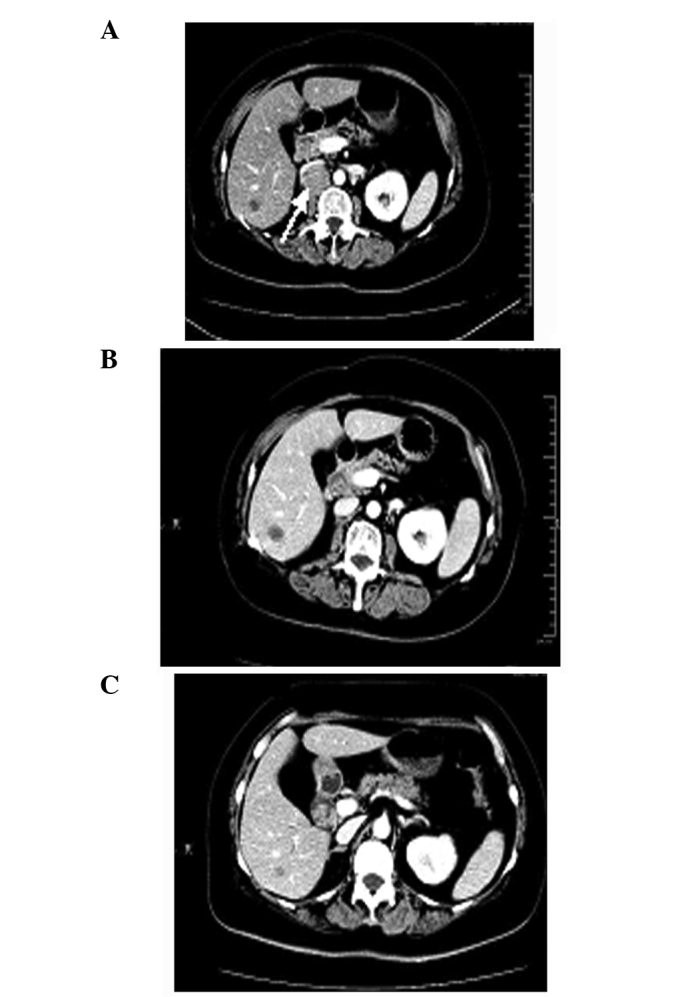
CT scan revealing (A) local recurrences in the retroperitoneum and (B) a considerably smaller tumor following chemotherapy with 80 mg/m^2^ intravenous irinotecan on days 1 and 8 and 25 mg/m^2^ cisplatin on days 1–3, every 21 days for 4 cycles. (C) The tumor was stable during the regular follow-up examinations. CT, computed tomography.
